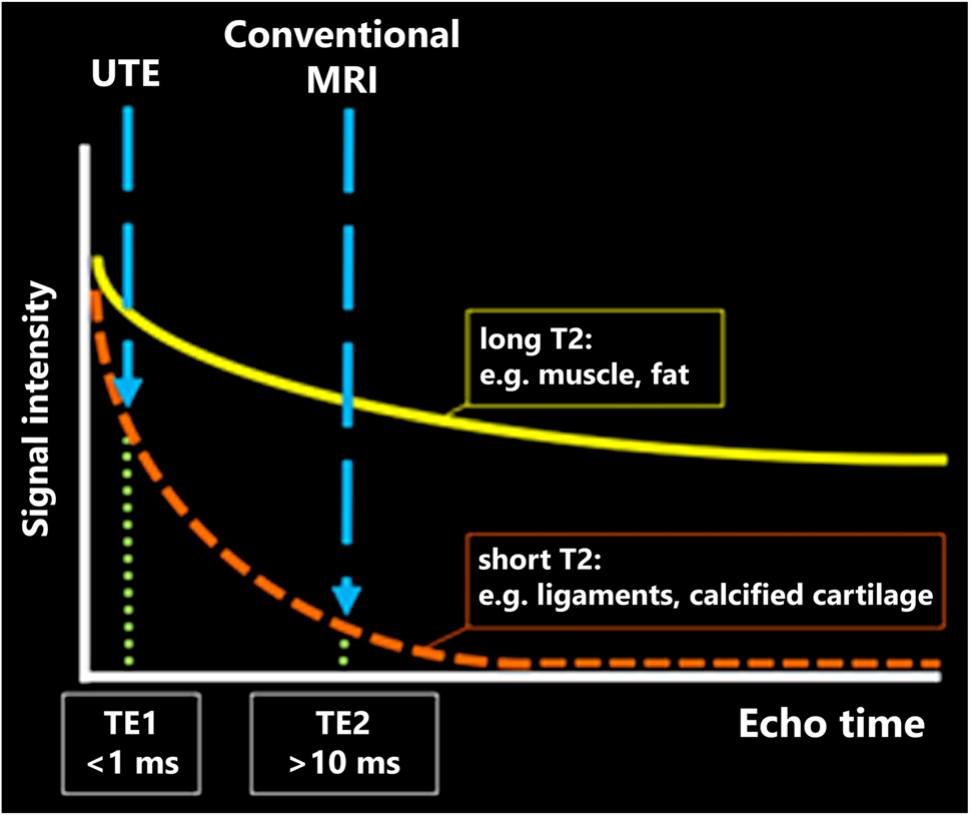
The decay of MR signal in tissues with different T2 properties is shown. In contrast to long T2 tissues like muscle and fat, in short T2 tissues like ligaments and calcified cartilage, the signal decays more rapidly. Using zero echo time and ultrashort echo time sequences featuring a very short TE (< 1 ms echo time), sufficient signal can be acquired. Abbreviations: TE = echo time; UTE = ultrashort echo time; MRI = magnetic resonance imaging
